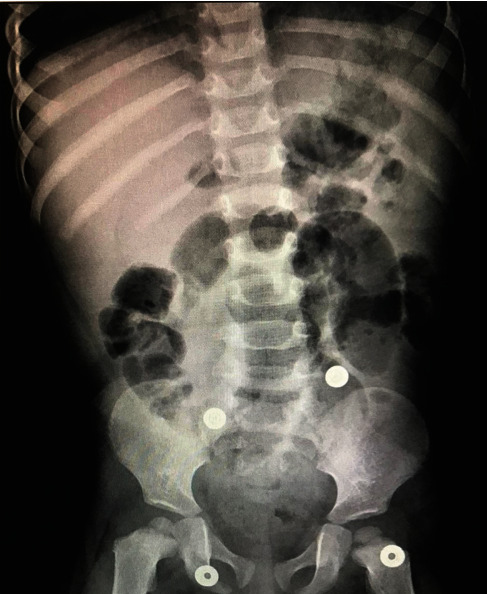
Postoperative abdominal X-ray shows resolution of the foreign body previously found preoperatively.
